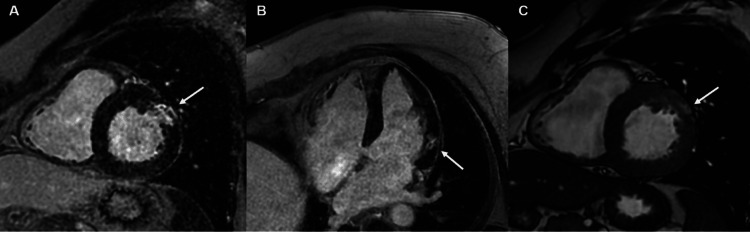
Cardiac magnetic resonance imaging Panel A: delayed gadolinium enhancement of the left ventricle in the short axis demonstrates a dense, transmural, focal area of hyperenhancement in the basal anterolateral segment with central hypoenhancement (arrow), consistent with myocardial infarct and microvascular obstruction. Panel B displays the infarct in a four-chamber view. Balanced steady-state free precession demonstrates hyperintense mid-myocardial signal relative to myocardium corresponding to the region of infarct, suggestive of lipomatous metaplasia (panel C, arrow)
